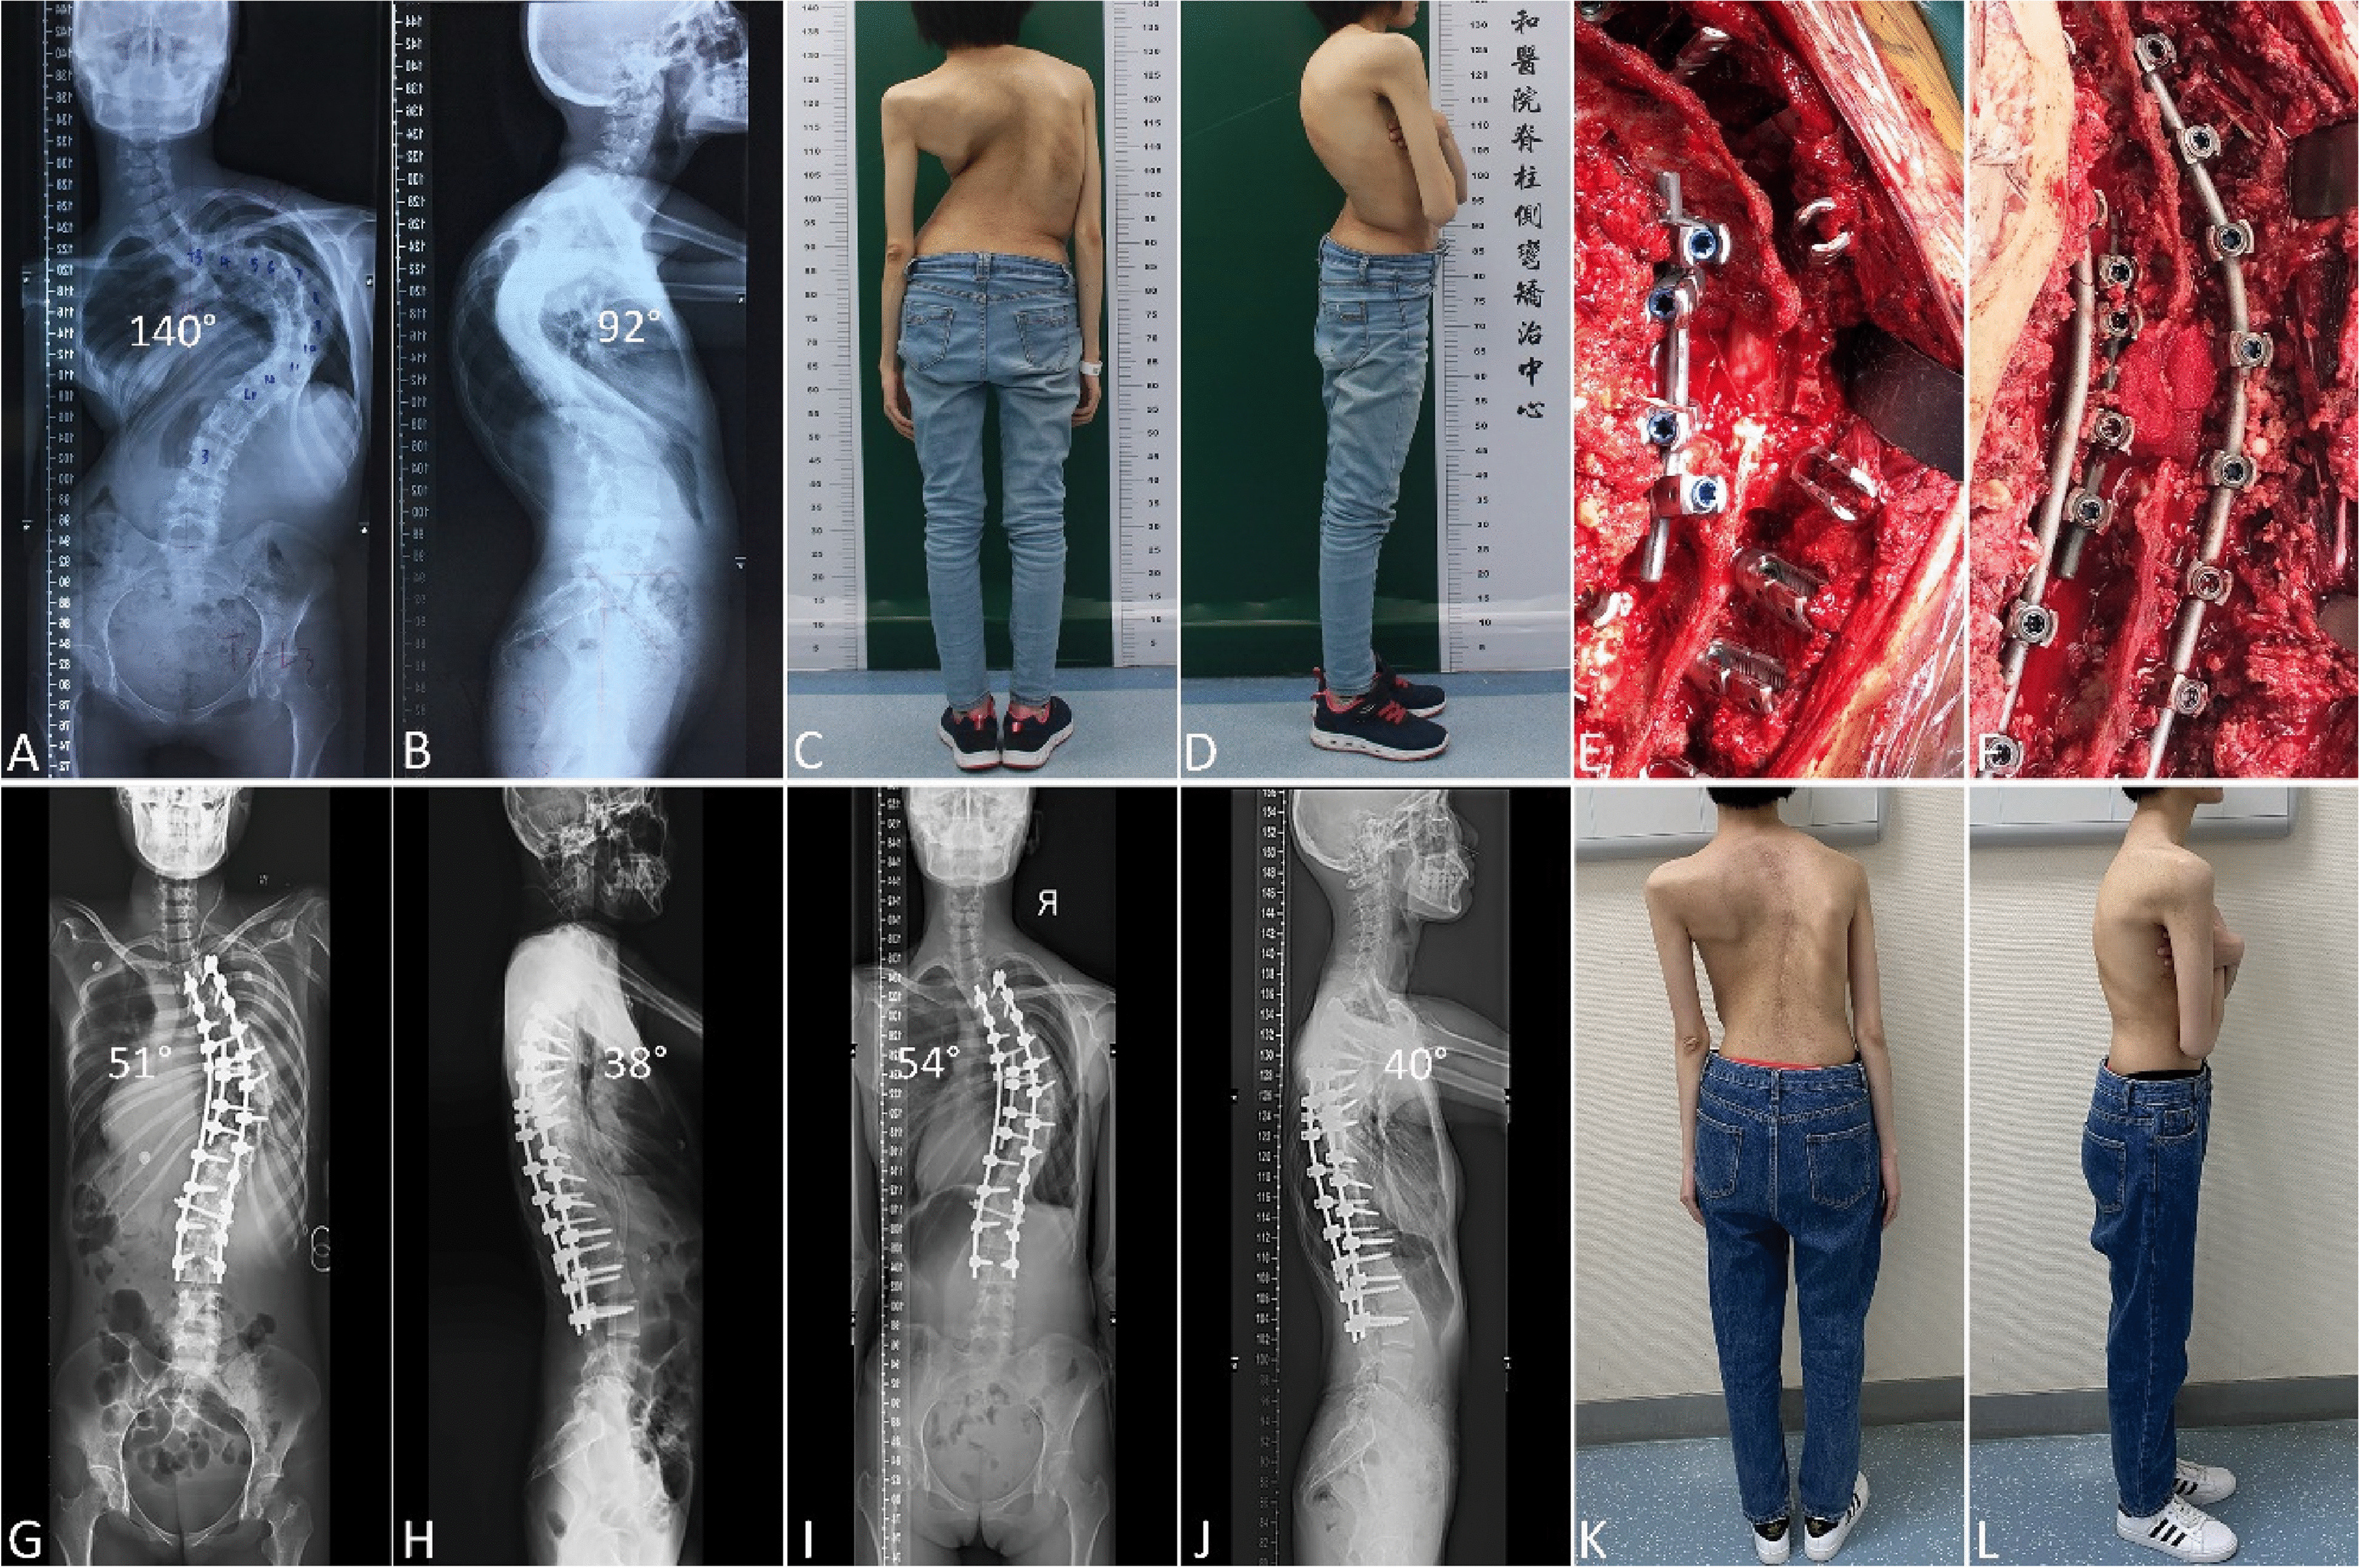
A 25-year-old woman with severe and rigid kyphoscoliosis treated by sequential correction combined with posterior grade 4 spinal osteotomy at the apical vertebrae. A, B, The coronal major curve angle and the kyphotic angle were 140° and 92° respectively on preoperative anteroposterior and lateral radiographs. C, D, Preoperative appearance. E, Grade 4 spinal osteotomy performed at the apical vertebrae (T9), and apical vertebrae correction maintained with short segmental instrumentation. F, Sequential correction completed. G, H, The coronal major curve angle and the kyphotic angle were 51° and 38° respectively on postoperative anteroposterior and lateral radiographs. I, J, The coronal major curve angle and the kyphotic angle were 54° and 40° respectively on postoperative anteroposterior and lateral radiographs at 24-month follow-up. K, L, Postoperative appearance
